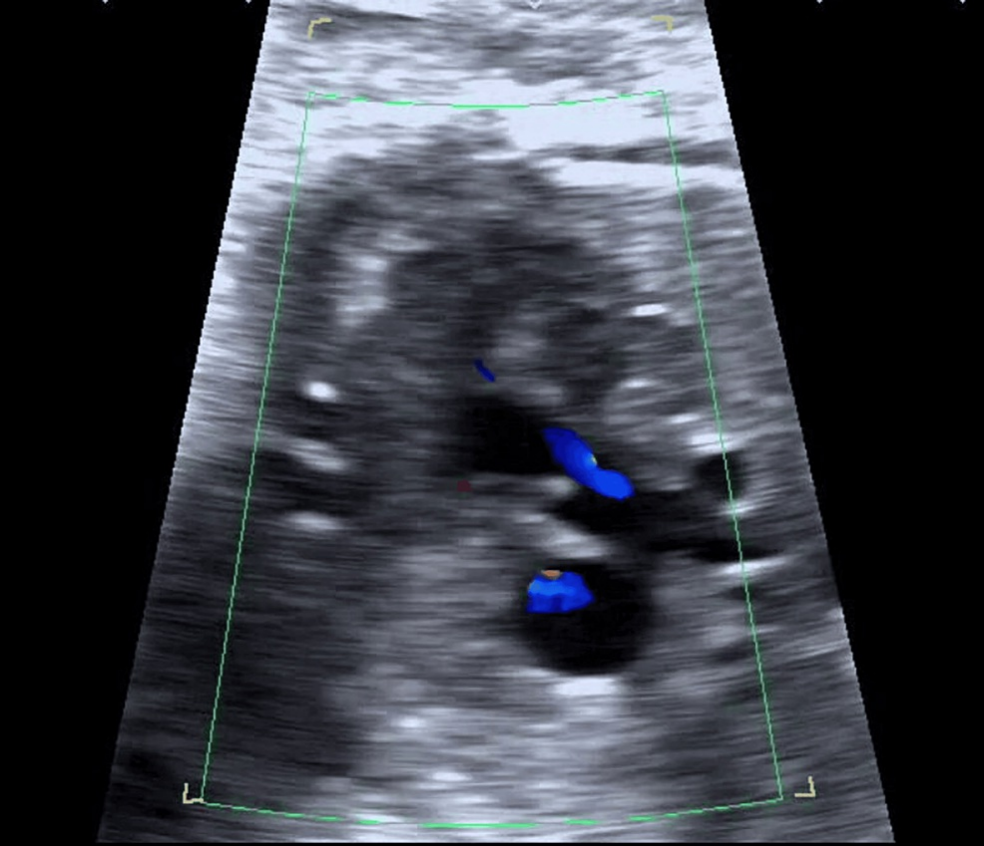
Echocardiogram showing severe pulmonary hypoplasia
